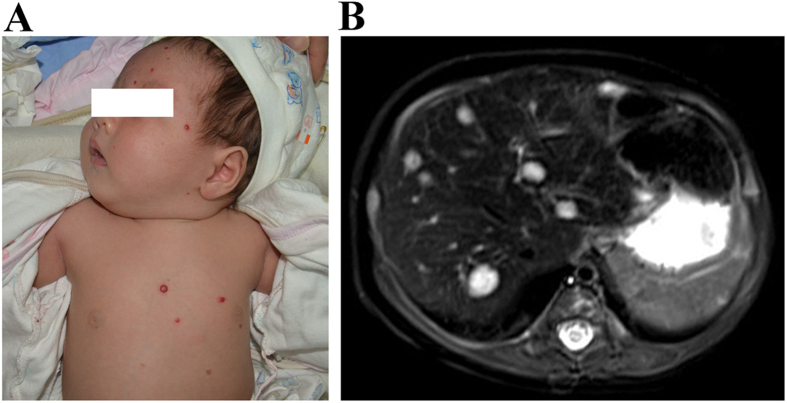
Multiple cutaneous IHs associated with MHHs in a 1-month-old girl. (A) Multiple IHs on the face and trunk. This patient also had additional lesions on the extremities. (B) T2-weighted axial MRI of the liver showed lesions were hyperintense with intervening areas of normal hepatic parenchyma.
